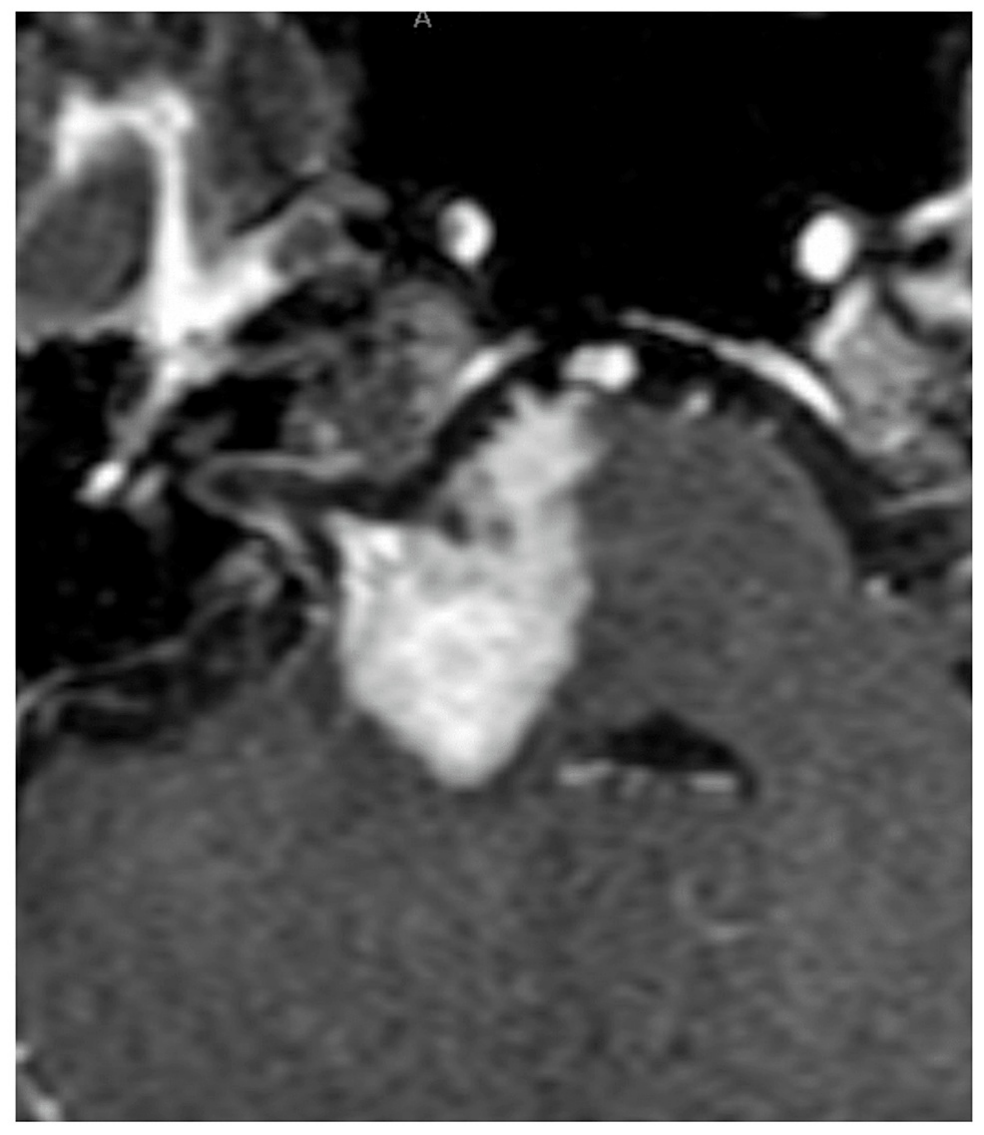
Contrast-enhanced T1-weighted MRI three months postoperatively MRI shows further enlargement of the tumor in the brainstem region, but no recurrence of tumor in the irradiated region. MRI: magnetic resonance imaging
